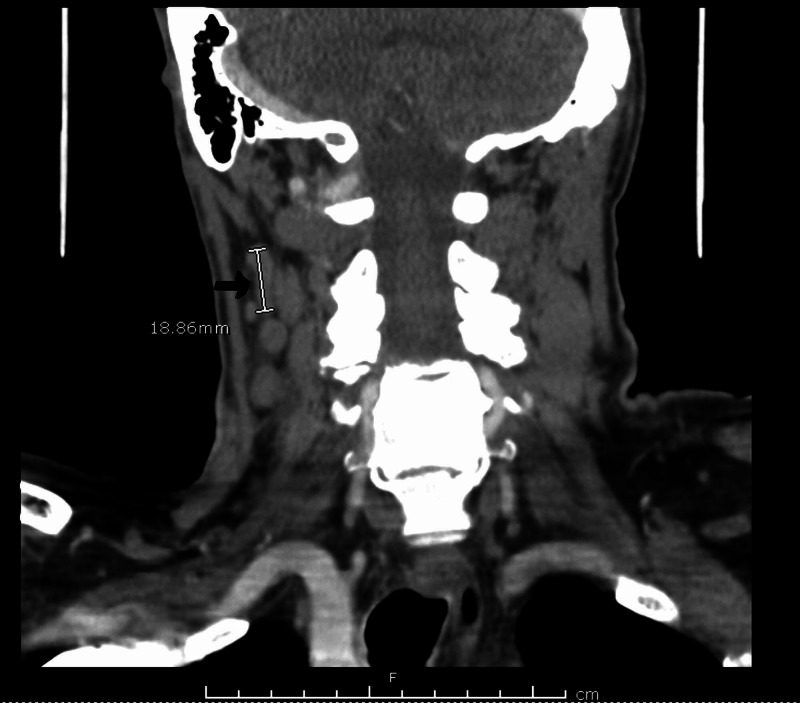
CT neck showing right cervical lymph node measuring 18.86 mm from which biopsy was obtained CT: computed tomography
